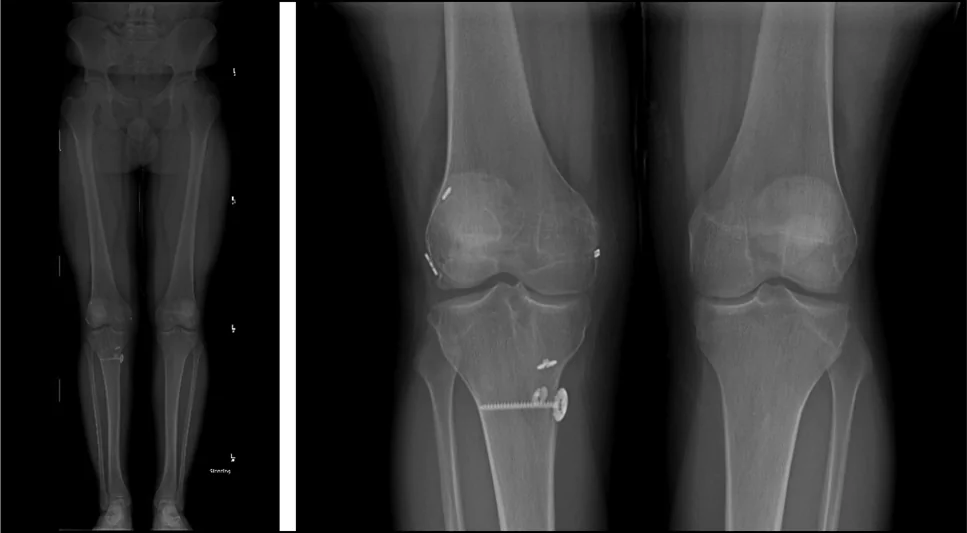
Standing leg length radiographs at 18 months postoperatively.
